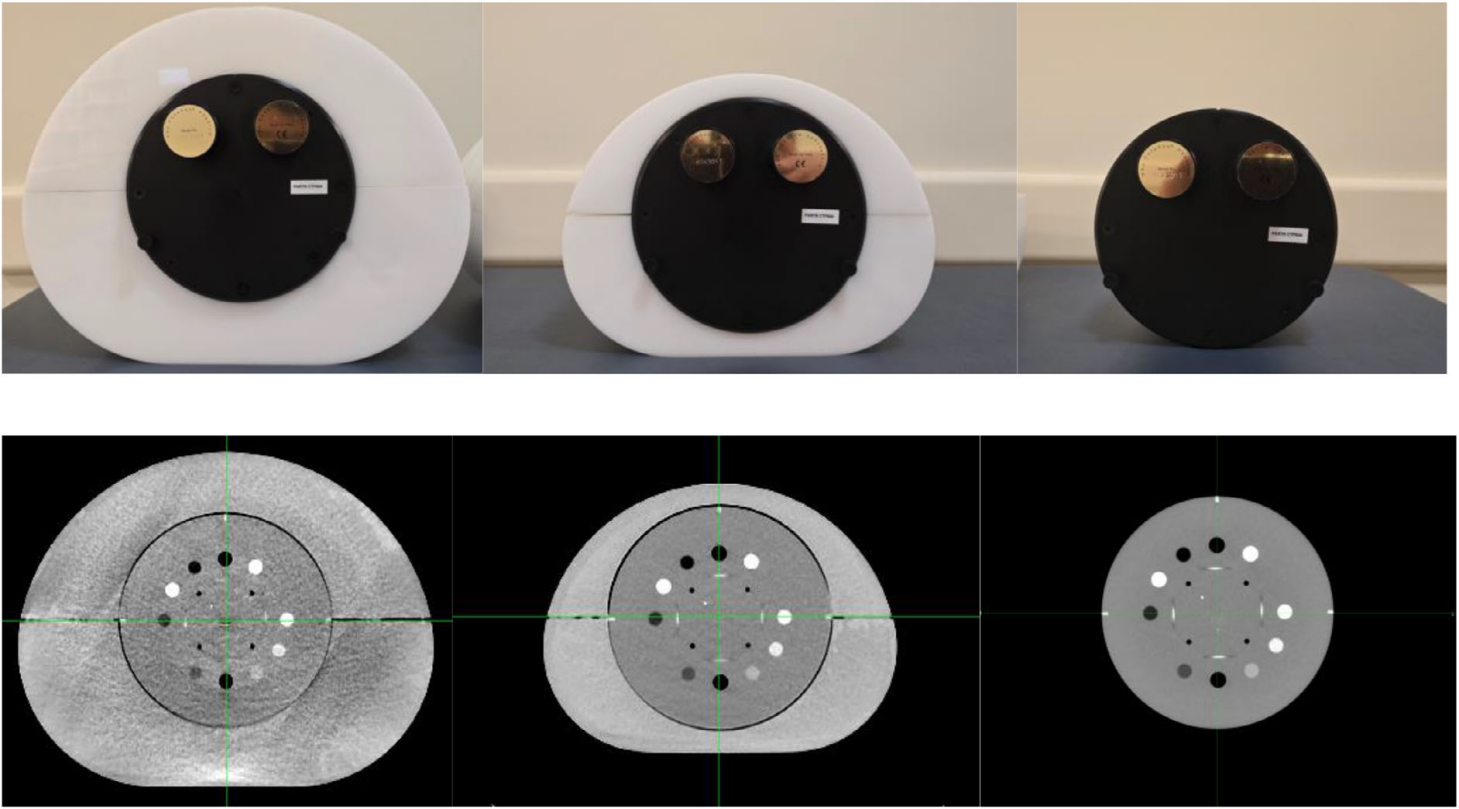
Top: CBCT scan setups showcasing the 40 cm annulus surrounding the CatPhan, the 32 cm annulus surrounding the CatPhan, and the CatPhan alone. Bottom: Axial slices corresponding to each CBCT scan setup.
